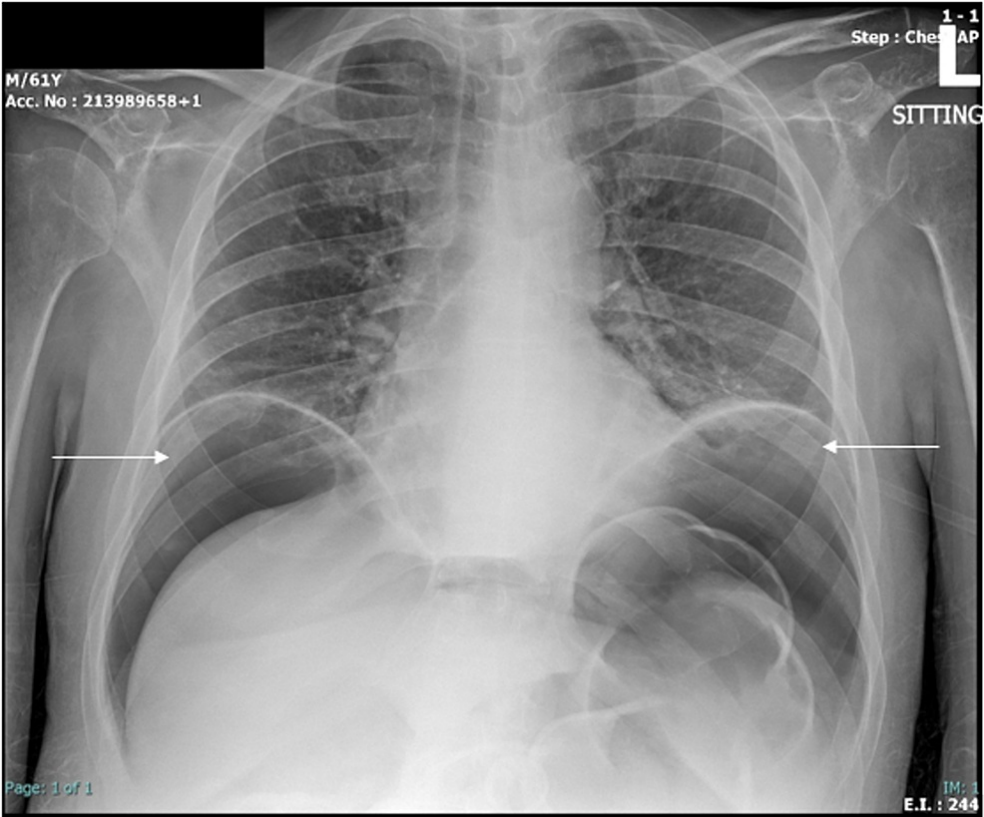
AP chest X-ray showing large amounts of subdiaphragmatic free air, bilaterally (arrows) AP: anteroposterior
